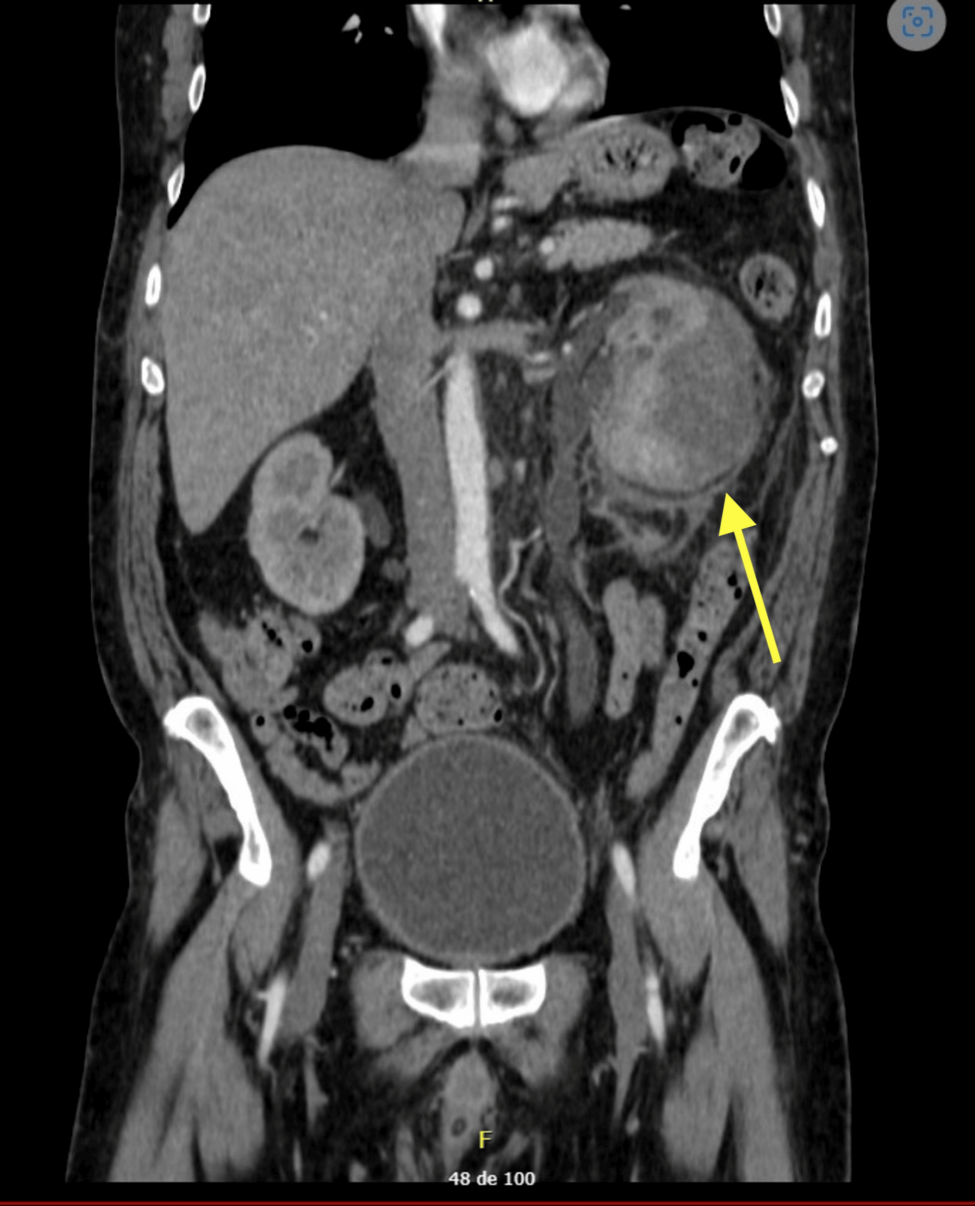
Axial CT showing a renal hematoma observed in the coronal section (yellow arrow) that displaces the renal parenchyma and causes hydronephrosis
